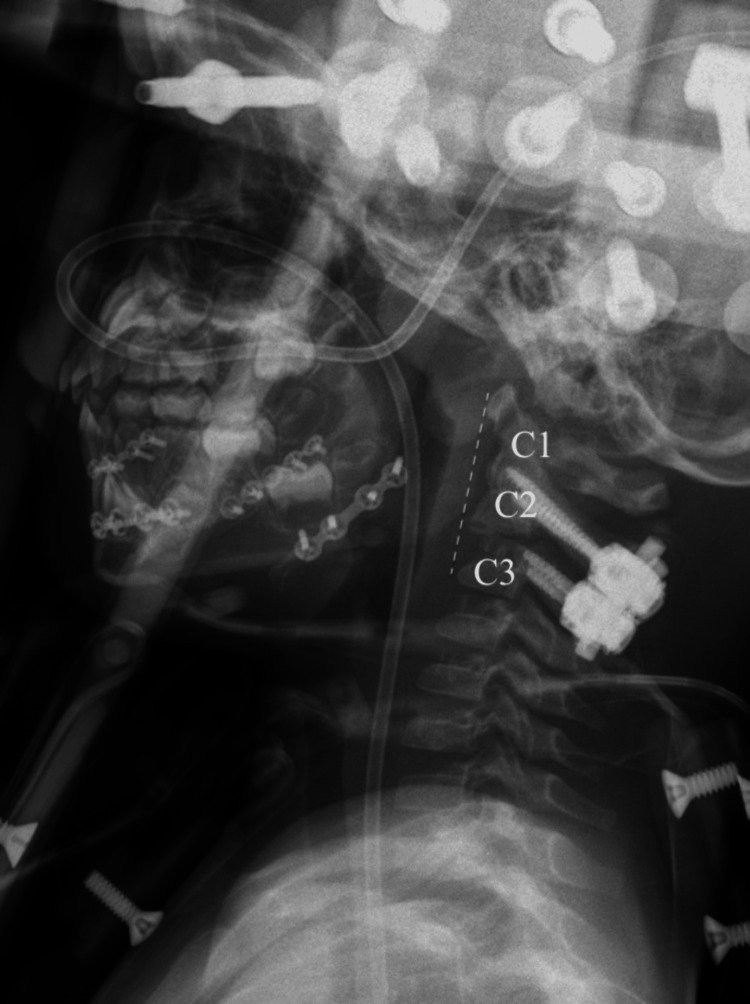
Lateral c-spine X-ray on hospital day 27 demonstrating continued satisfactory alignment of cervical spine following posterior fusion at C2-C3.
